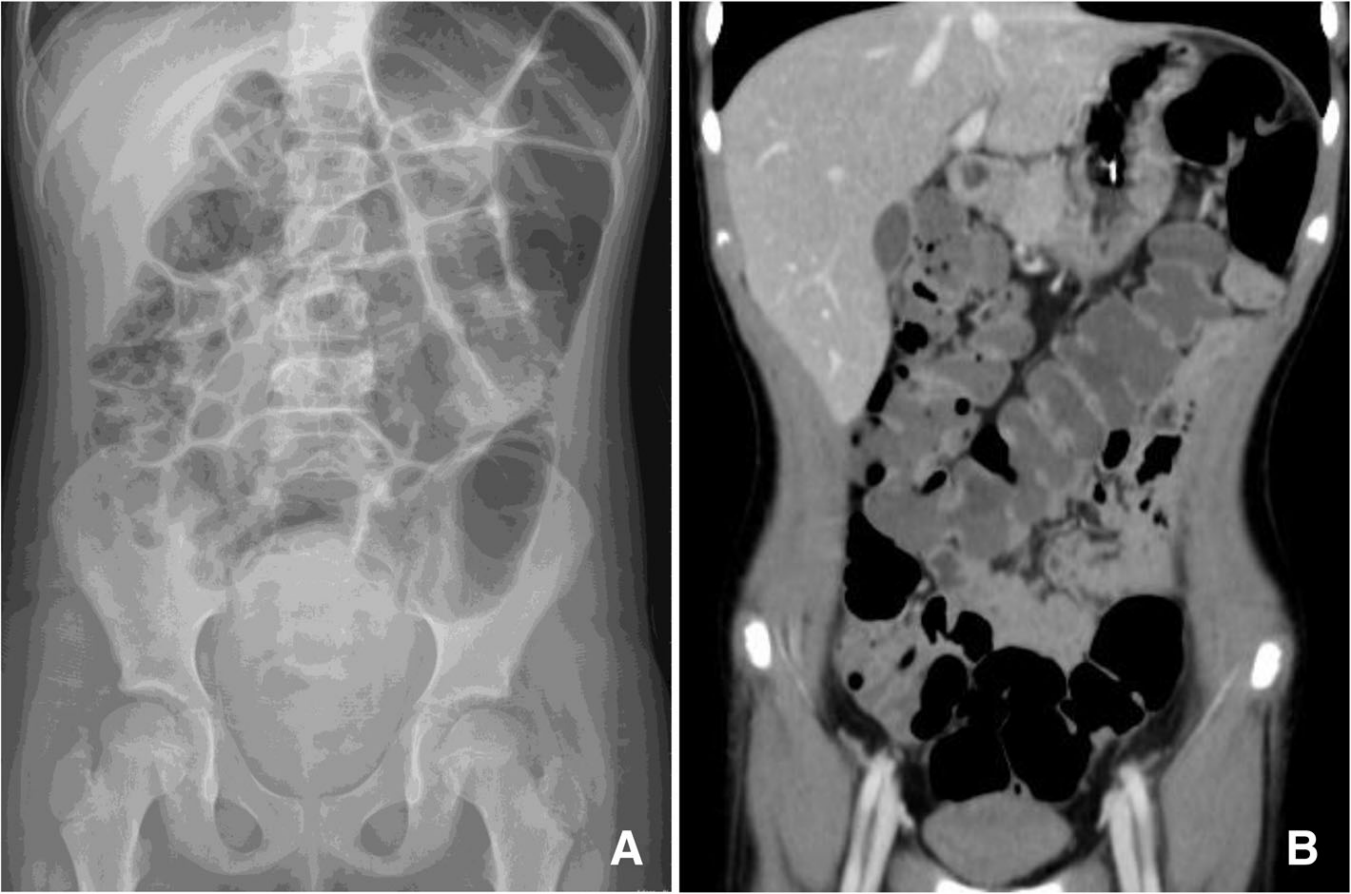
a Anterioposterior abdominal x-ray showing marked dilatation of the colon, without air/fluid levels and free air. b Coronal computed tomography revealed massive dilatation of the large bowel with minimal involvement of the small bowel
